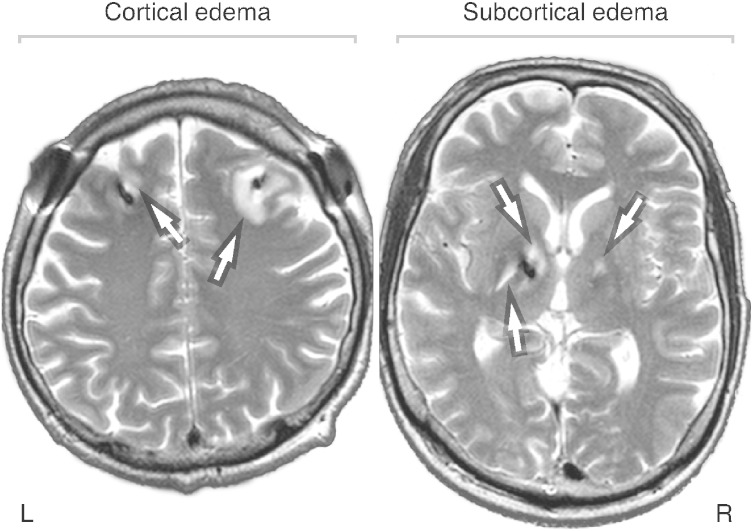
T*_*2*_-weighted MR images demonstrating collateral edema caused by the DBS electrode insertion at the cortical (left panel; rating 5; patient 7) and subcortical levels (right panel; rating 3.5; patient 8). The edema appears as hyperintensity (indicated by arrows), while the DBS electrode artifacts are hypointense. MR — magnetic resonance; DBS — deep-brain stimulation; L — left and R — right.
